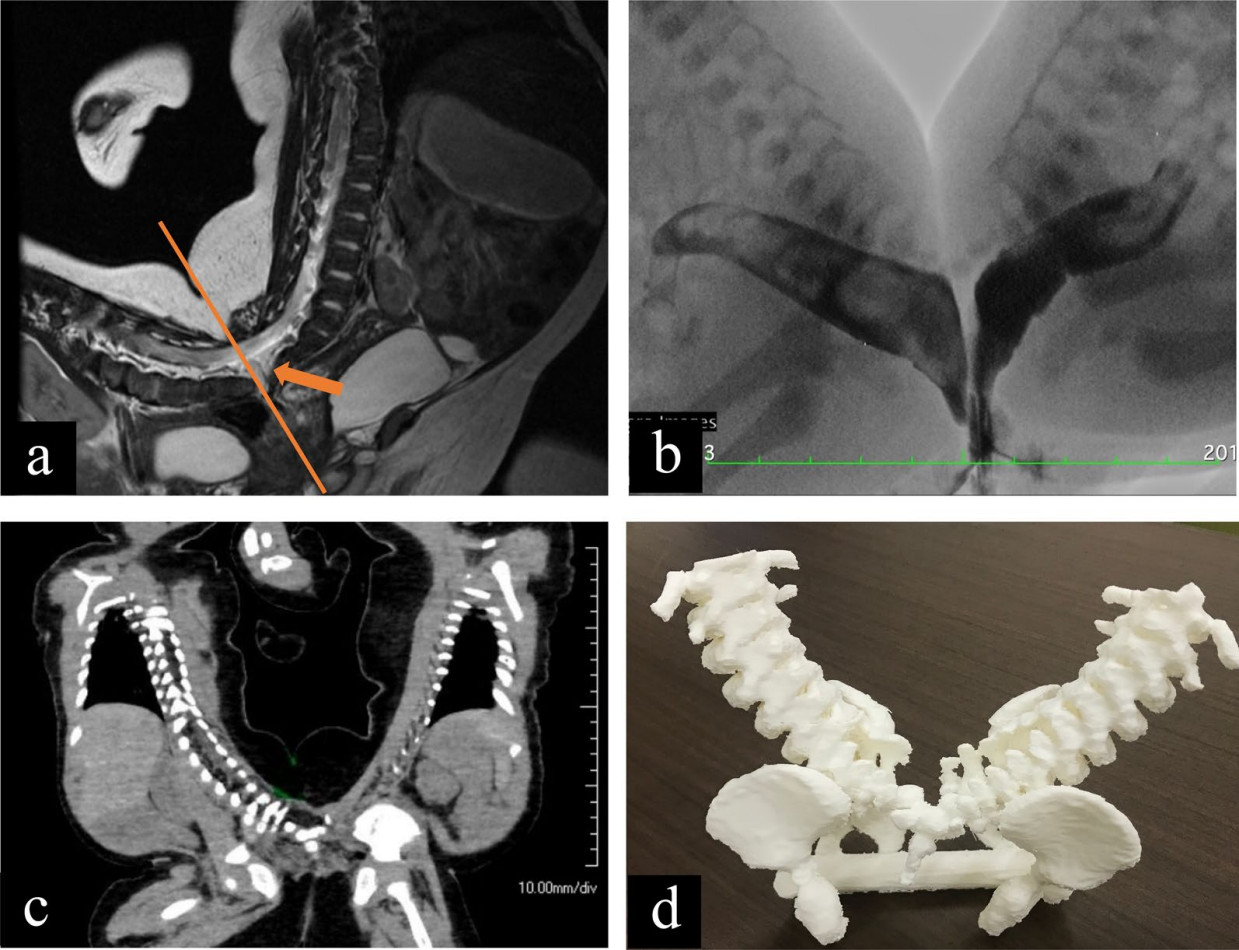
Preoperative image findings and the 3D model. Preoperative image of a a fused spinal cord detected by MRI (arrow), b separated rectums detected by a gastro-graphin enema. c Other intra-abdominal organs have no connection, d the 3D model was created for multidisciplinary discussion
